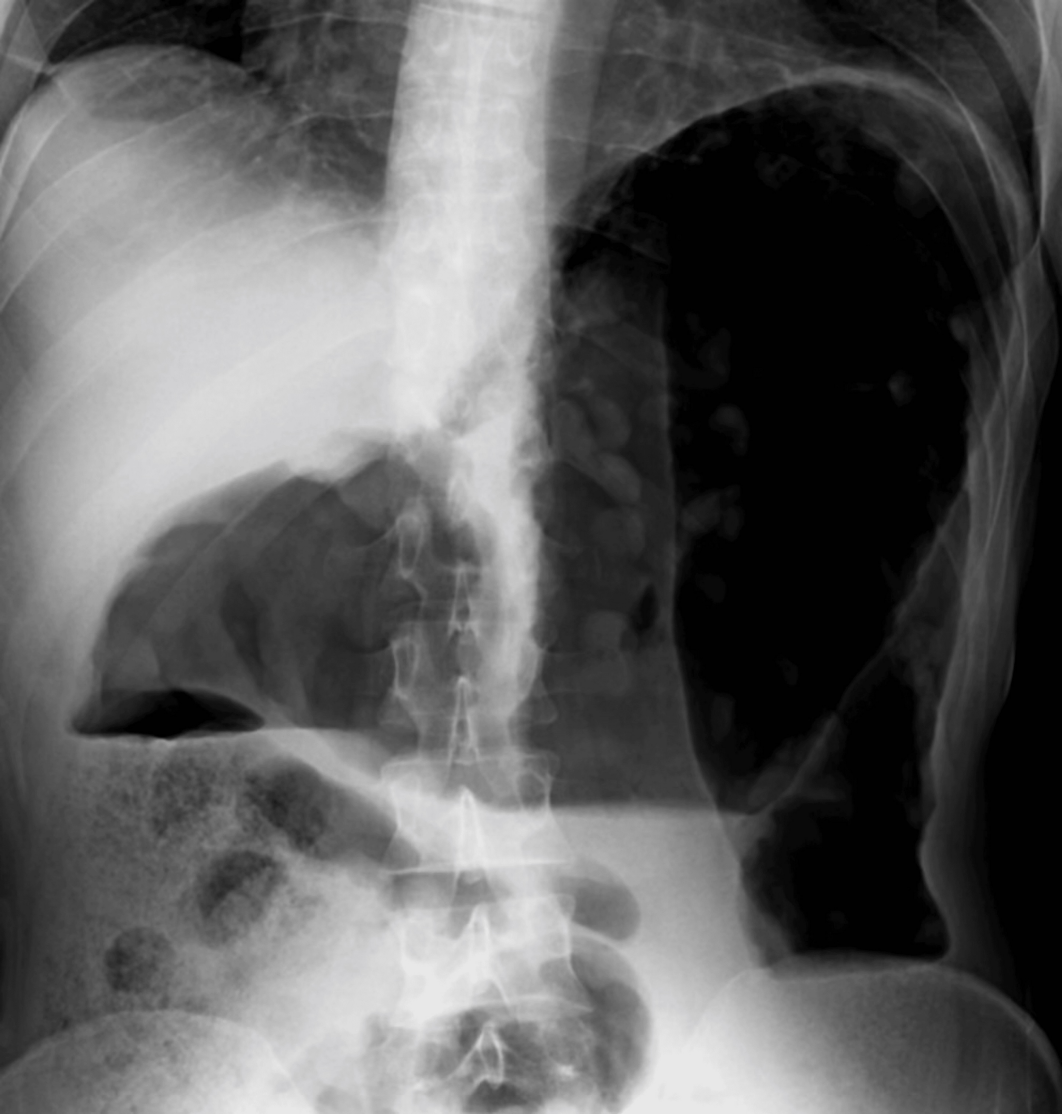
Abdominal radiograph demonstrating marked colonic dilation and loss of haustral markings, consistent with toxic megacolon
